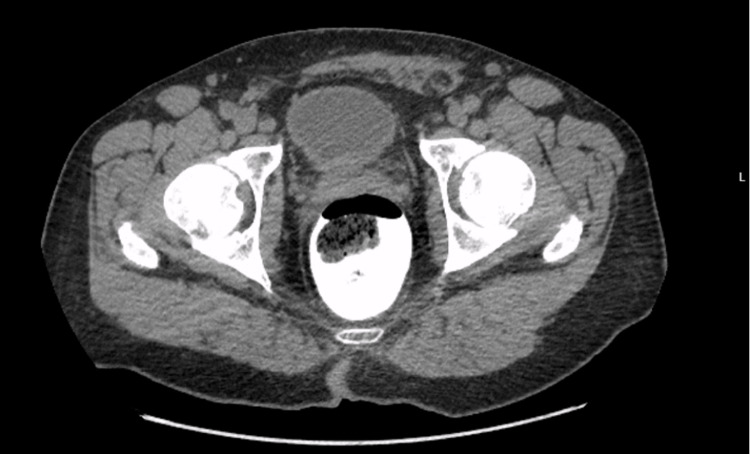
Computed tomography of the abdomen/pelvis with rectal contrast Taken four months after index case, the image demonstrates marked resolution of soft tissue phlegmononous changes.
